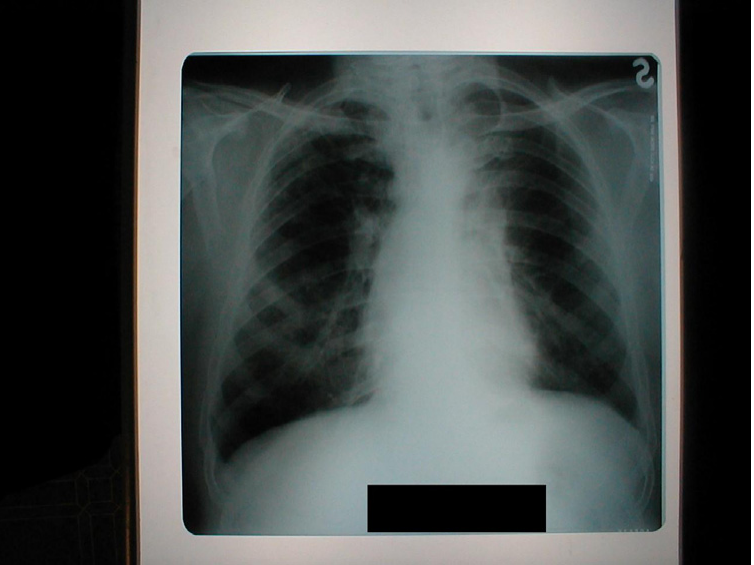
X-ray of the thorax. Infiltrative changes are observed in the left upper lobe.
